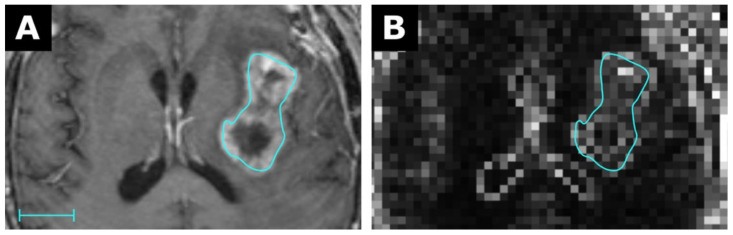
MRI of a glioblastoma (A) with a corresponding map for the feature Robust Mean Absolute Deviation (RMAD) with a resolution of 3 × 3 × 3 mm in (B). Scale indicates 2 cm. The value for RMAD from the conventional extraction was 42.3 and 18.0 for the map. Details are available in electronic Table S3.
